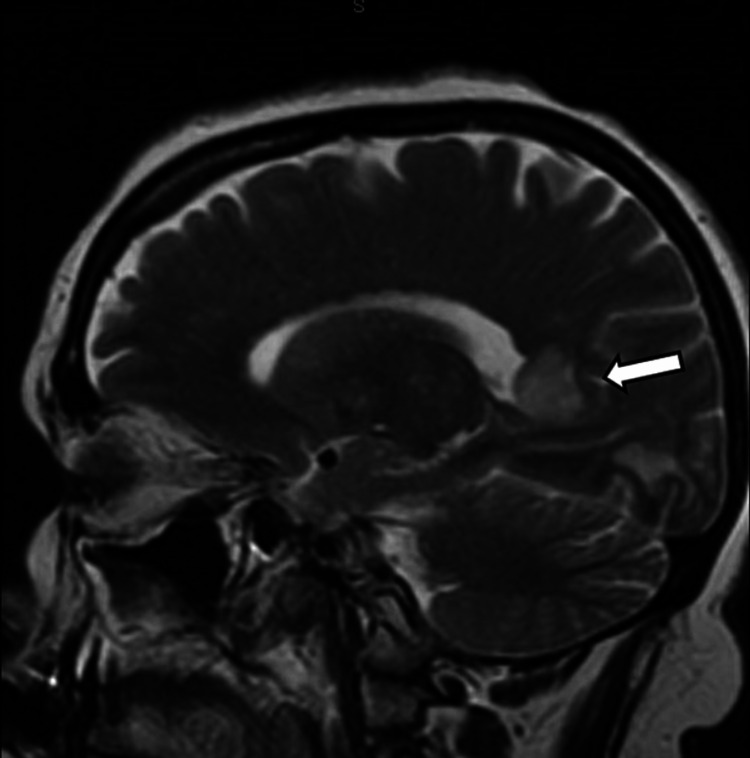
MR brain with contrast. Sagittal view of MR brain showing increased T2/FLAIR signal in the periventricular and subcortical white matter. MR: magnetic resonance, FLAIR: fluid-attenuated inversion recovery.
